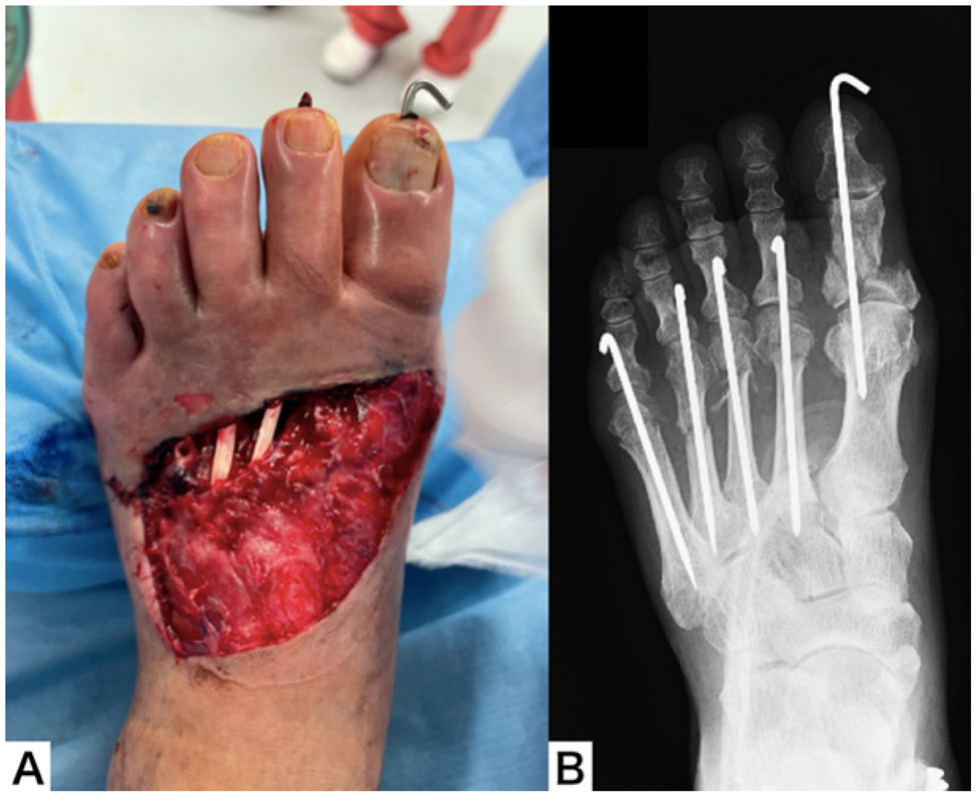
Appearance (A) and plain radiograph (B) of the left foot after Emergency operation.
